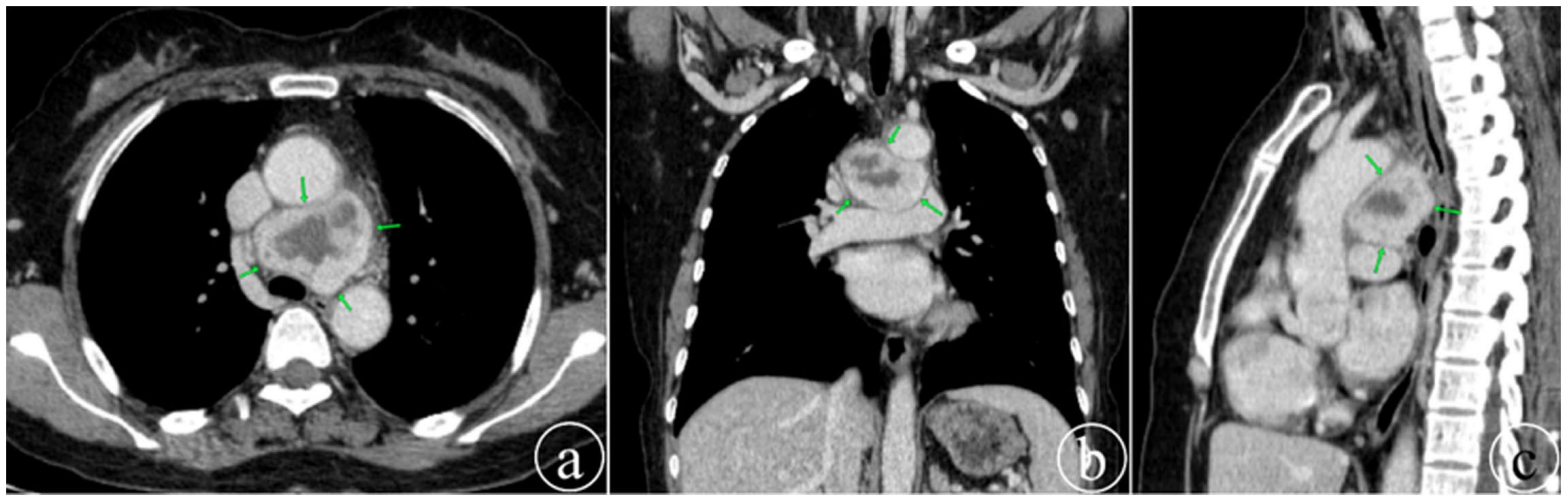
Contrast-enhanced chest CT during the initial visit shows a mediastinal mass in the venous phase (arrow). Panels (a–c) display axial, coronal, and sagittal views, respectively, revealing heterogeneous density of the mass and its close relationship with the heart and great vessels.
